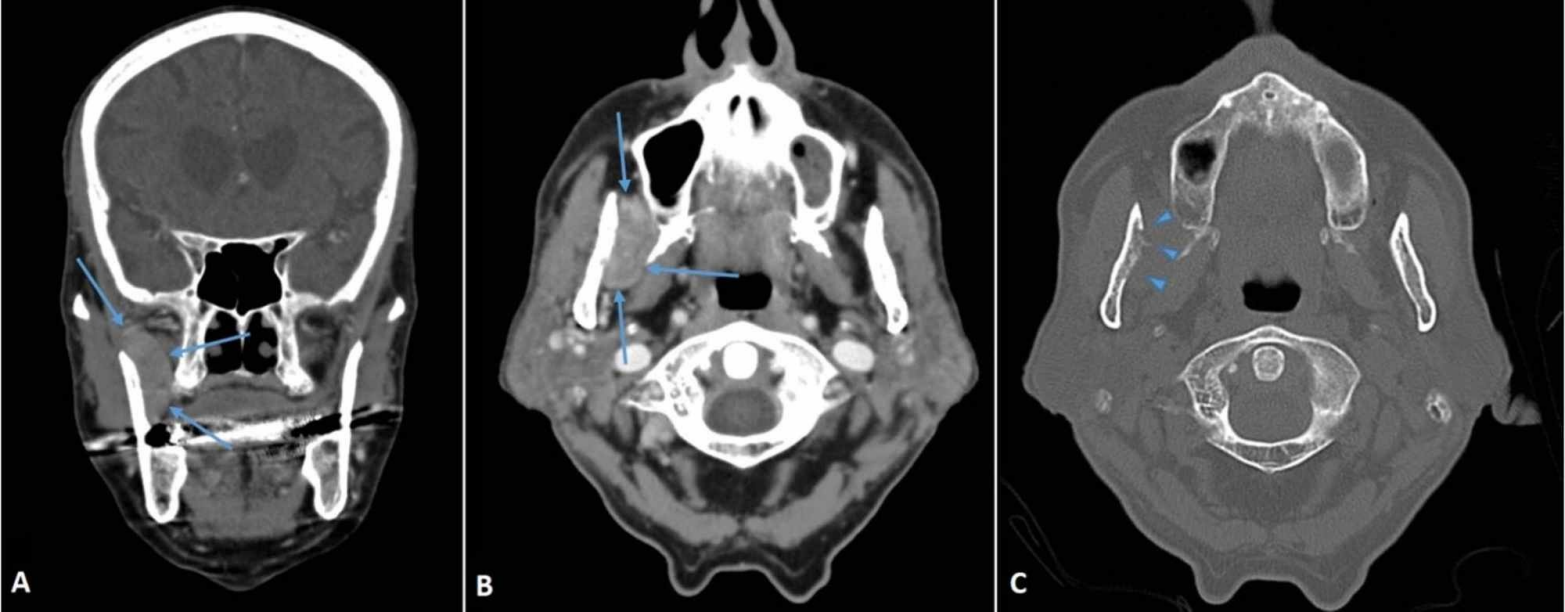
Masticatory space metastasis. (A, B) CT scan of the neck showing a mass in the right masticatory space; lateral to the lateral pterygoid muscle, and medial to the ramus of the mandible (arrows). Also, partial destruction of the mandible (C) is visible (arrowheads).
